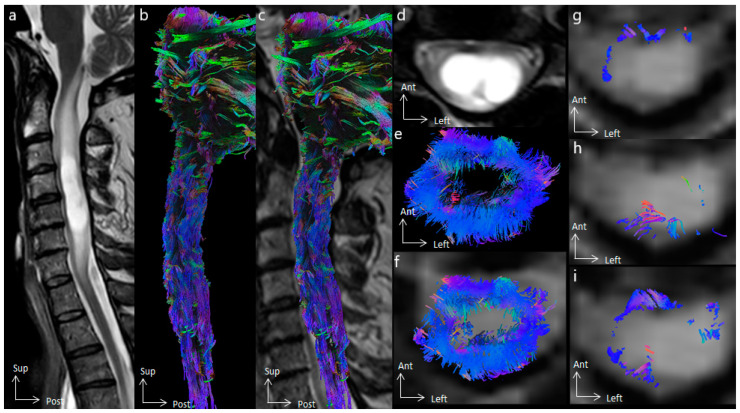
Spinal cord tractography in patient 5, with an ependymoma. (a) Sagittal T2-weighted image showing cystic C3–C4 intramedullary tumor, whose cystic walls are enhanced after gadolinium injection. (b) Lateral view of the spinal cord tractography. (c) Overlay of the spinal cord tractography on the sagittal T2-weighted MR images, suggesting lateral loss of fibers at the C3 spinal level. (d) Axial T2-weighted images showing a doubled-lobed cystic tumor. (e) Axial view of spinal cord tractography at the C4 level. (f) Overlay of the spinal cord tractography on the axial T2-weighted MR images, showing that spinal fibers were compressed by the tumor on the surface of the spinal cord, and suggesting ependymoma. (g) Overlay of the corticospinal tract on the axial T2-weighted MR images, compressed ventrally. (h) Overlay of the dorsal columns on the axial T2-weighted MR images, compressed dorsally, and within the tumor on the left side. (i) Overlay of the spinocerebellar tracts on the axial T2-weighted MR images.
